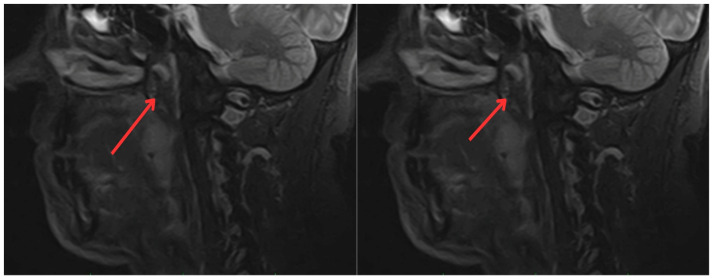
Contrast-enhanced magnetic resonance imaging of the neck: irregular enhancement, forming a mass that involves the mucopharyngeal space and the right palatine tonsil, extending to the tongue base, with a neoplastic appearance (red arrows).
